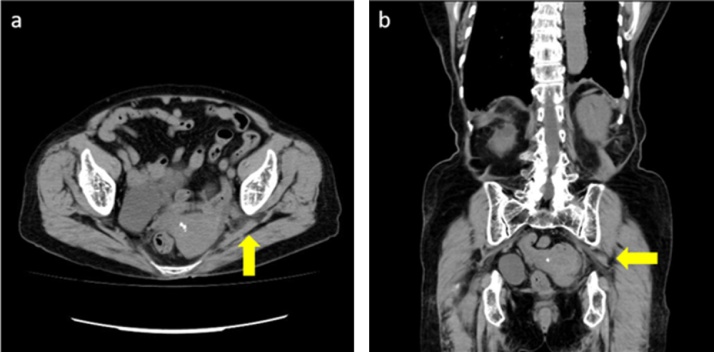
Post-procedure unenhanced abdominal computed tomography scan confirmed reduction of the left ureter (arrow). a. axial view, b. coronal view.
